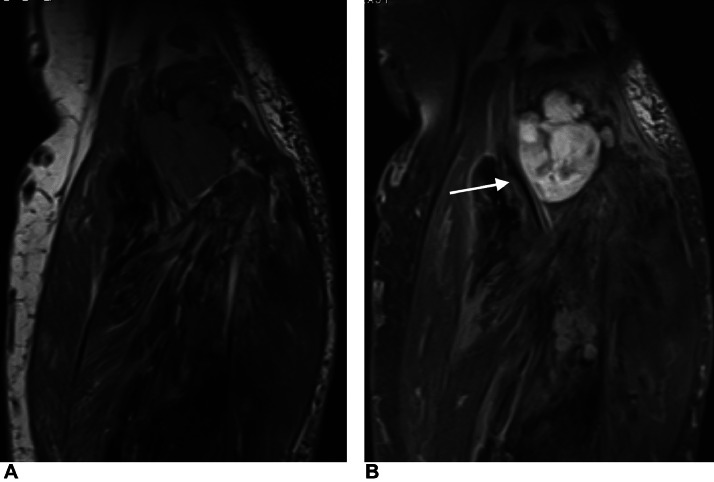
MRI image, coronal T1 tse (A), T1 tse FS (B) after gadolinium administration. Images show various levels of the contrast medium uptake in the hypointense, well-demarcated soft-tissue areas of the mass (arrow).
